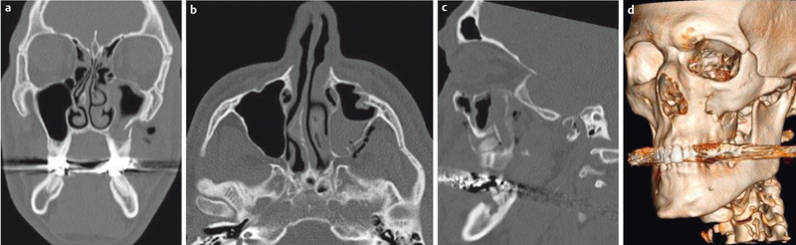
19-year-old patient who fell on the face. CT of the midface in bone window-level setting (a–c) demonstrates the exact extent of the complex fracture of the maxillary sinus, zygomatic arch (“tripod fracture”), and the floor of the orbit. 3D-VRT reconstruction demonstrates the anatomical relationship of the different fragments.
